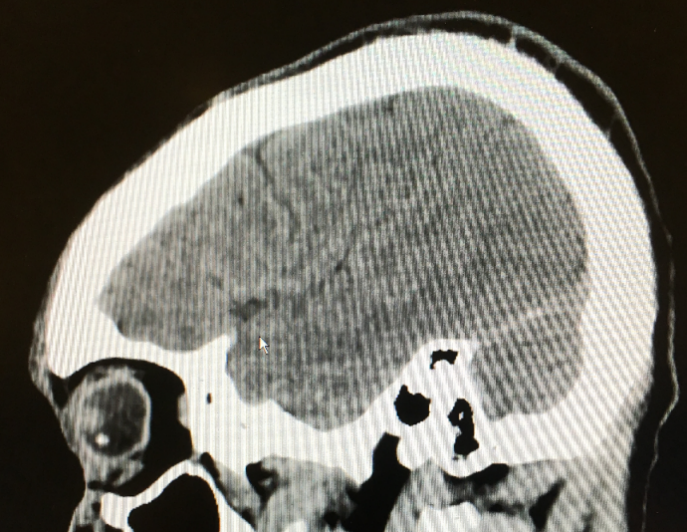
A foreign body seen within the globe on CT-imaging
